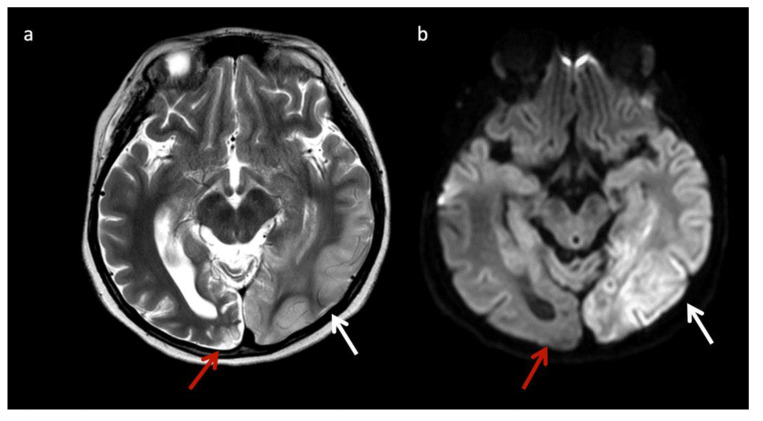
Eleven-year-old male with new onset seizures and history of multiple stroke-like episodes attributed to mitochondrial encephalopathy, lactic acidosis, and stroke-like episodes (MELAS). (a) Axial T2WI and (b) axial DWI at the level of the midbrain show nonterritorial cortical/subcortical edema/swelling in the left occipito-temporal region involving both middle and posterior cerebral artery territories consistent with acute metabolic injury (white arrows). Volume loss, T2 prolongation, and facilitated diffusion consistent with encephalomalacia from old metabolic injury is noted in the right occipito-temporal region (red arrows).
